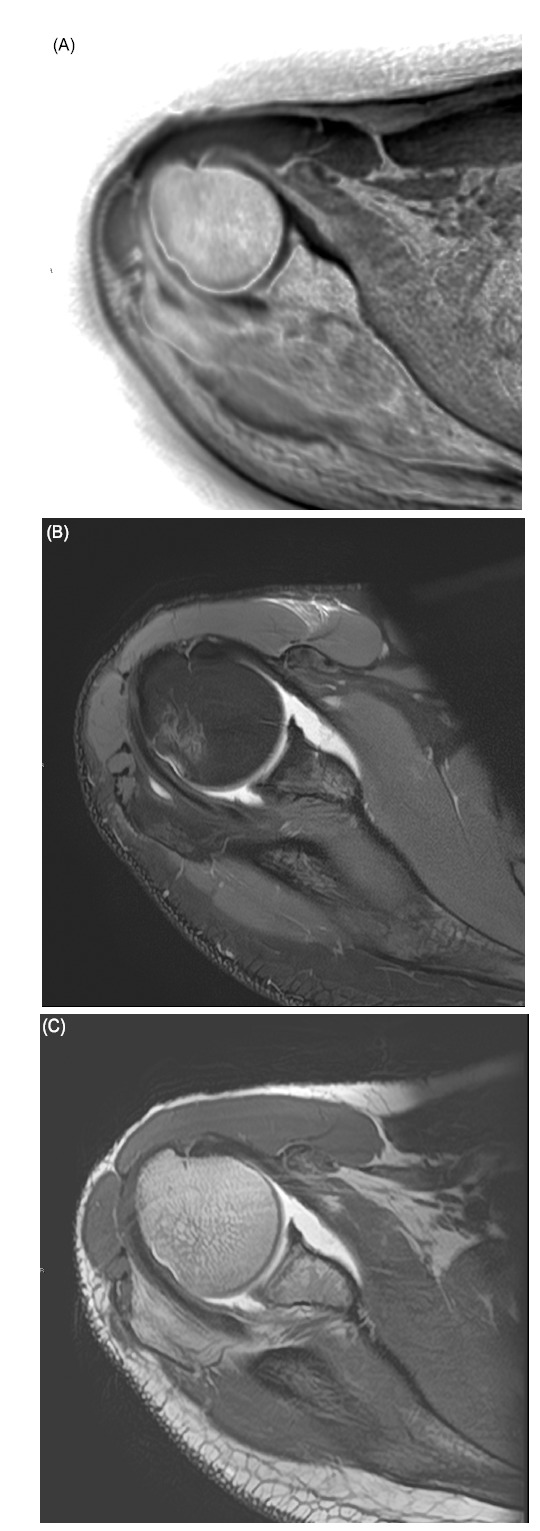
UTE MRI technique. A 22-year-old patient presented with a recent history of shoulder dislocation. The UTE image (A) delineates a small bone deformity at the posterior humeral head, compatible with a Hill-Sachs lesion. Axial FS PDWI (B) and T1WI (C) images are included for comparison.
